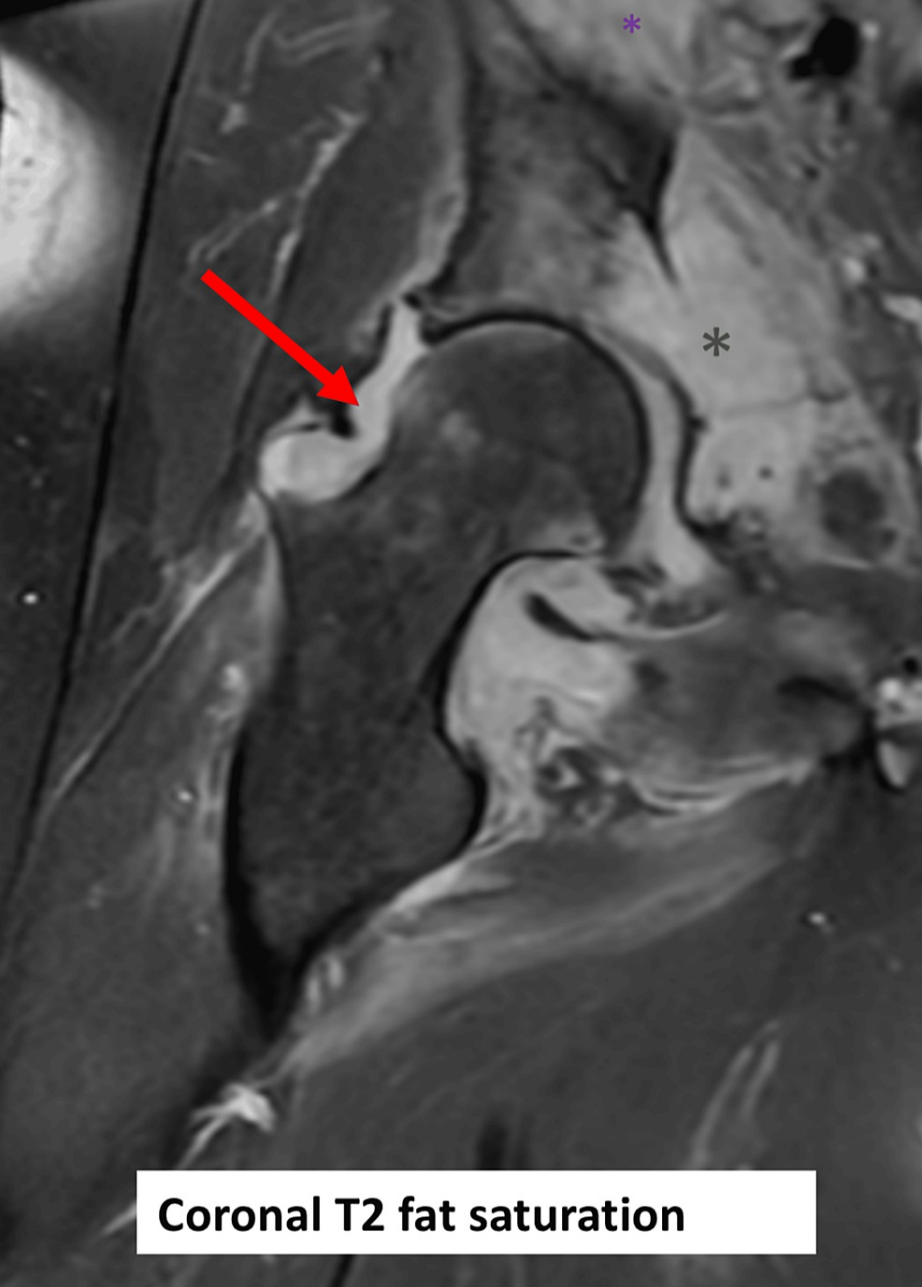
Right hip MRI (coronal T2 fat saturation). Right-sided joint effusion (red arrows) with acetabular destruction (green asterisk).
